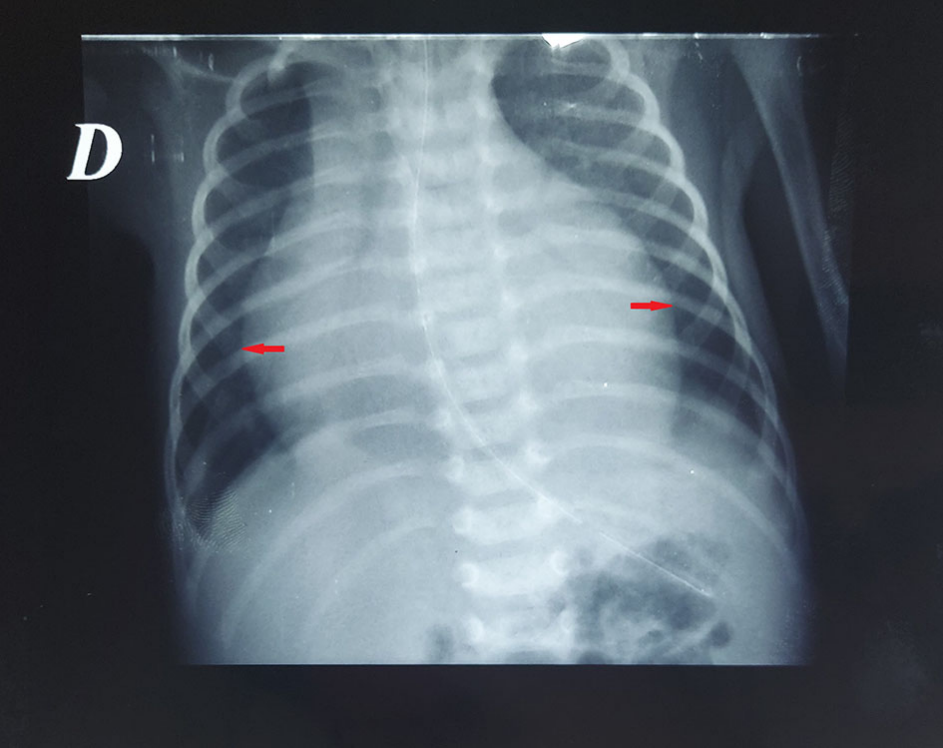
Pre-pericardiocentesis chest radiography demonstrating marked cardiomegaly with globular cardiac silhouette.
